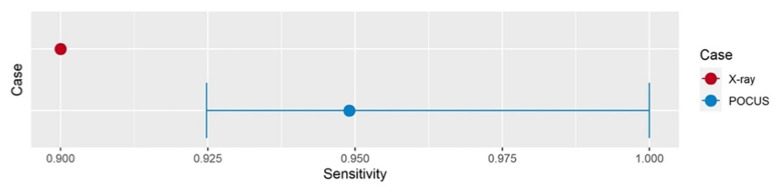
One-sided confidence interval of POCUS vs. X-ray imaging.
